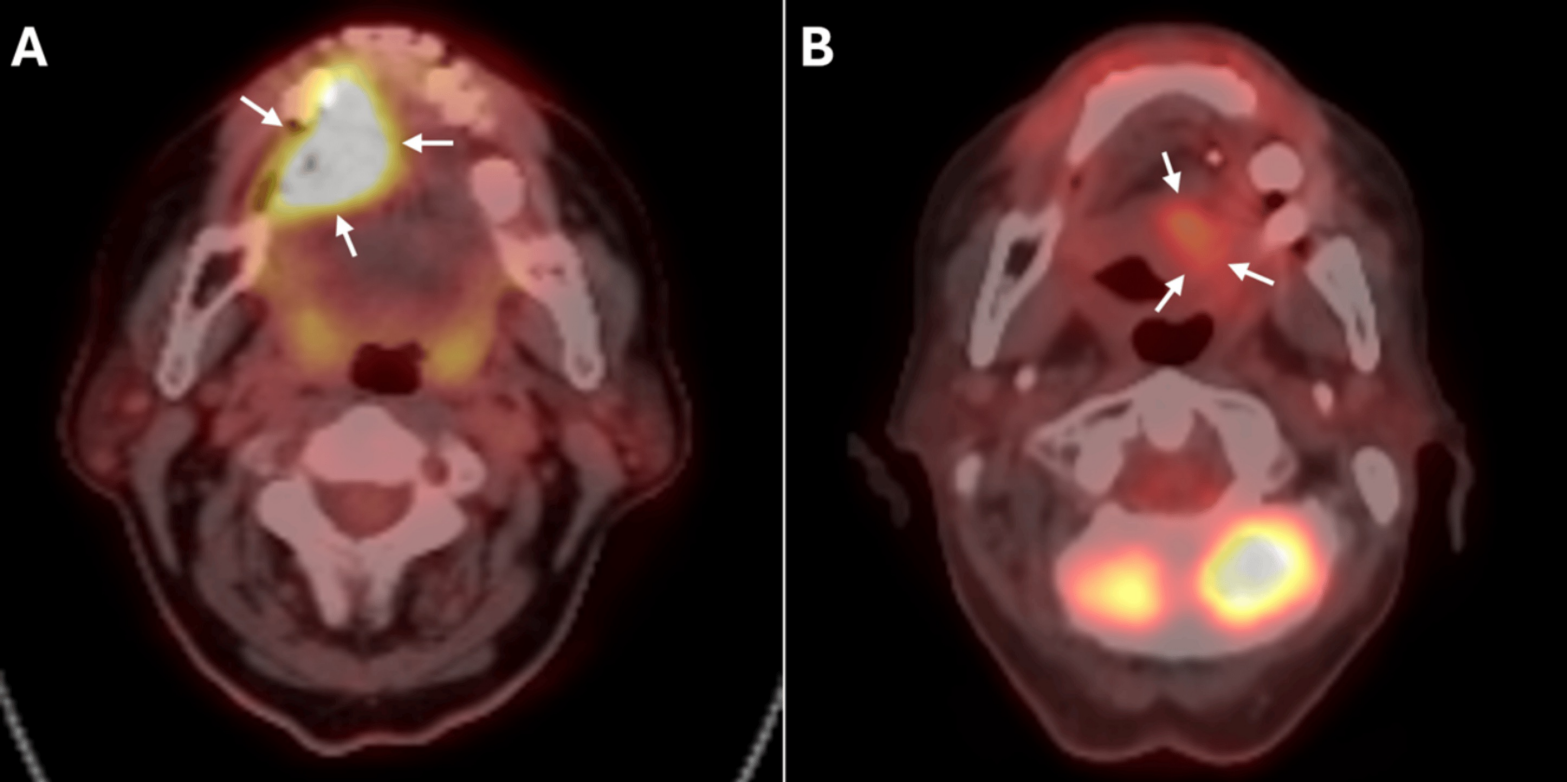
Preoperative and post-radiotherapy PET/CT findings Preoperative PET/CT showing a hypermetabolic primary tongue mass (A) and postoperative PET/CT at three months demonstrating mild metabolic activity at the surgical site consistent with post-treatment changes (B). White arrows indicate areas of FDG uptake.
